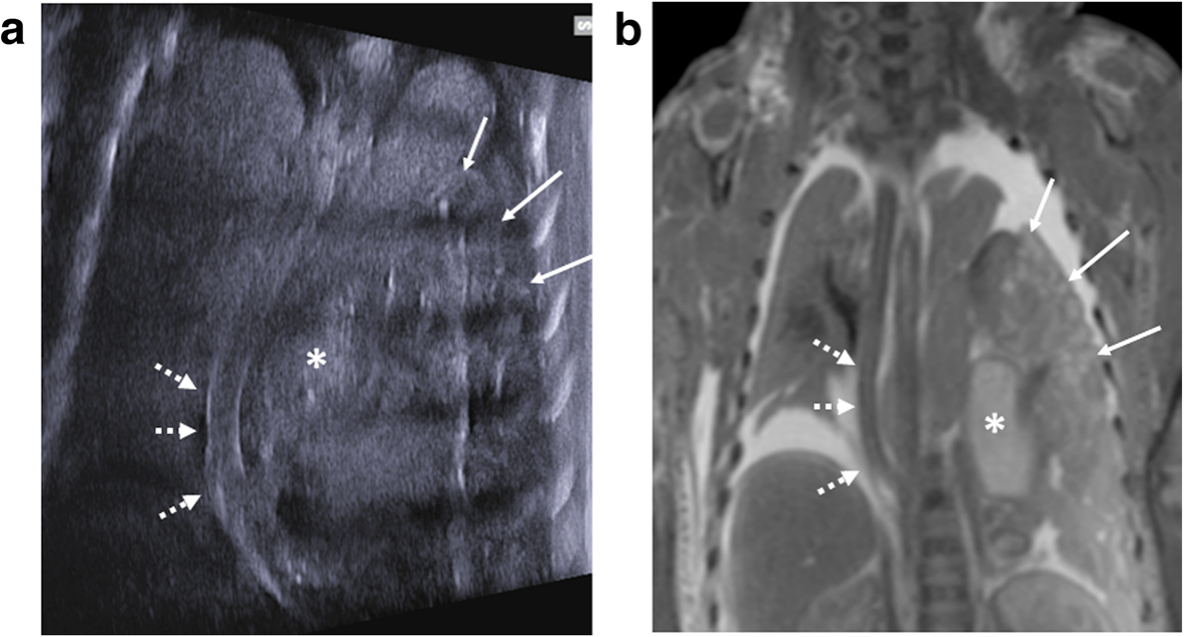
Large left sided congenital diaphragmatic hernia in a foetus at 24 weeks gestation, following termination of pregnancy. Post-mortem ultrasound and MRI were performed 24 hours apart, 12 days after death. Coronal plane imaging of the thorax at post-mortem ultrasound (a) and post-mortem T2 weighted MRI at 1.5T (b) show herniated bowel loops (solid white arrows), herniated stomach (asterisk) and the deviated course of the oesophagus (dotted white arrows)
